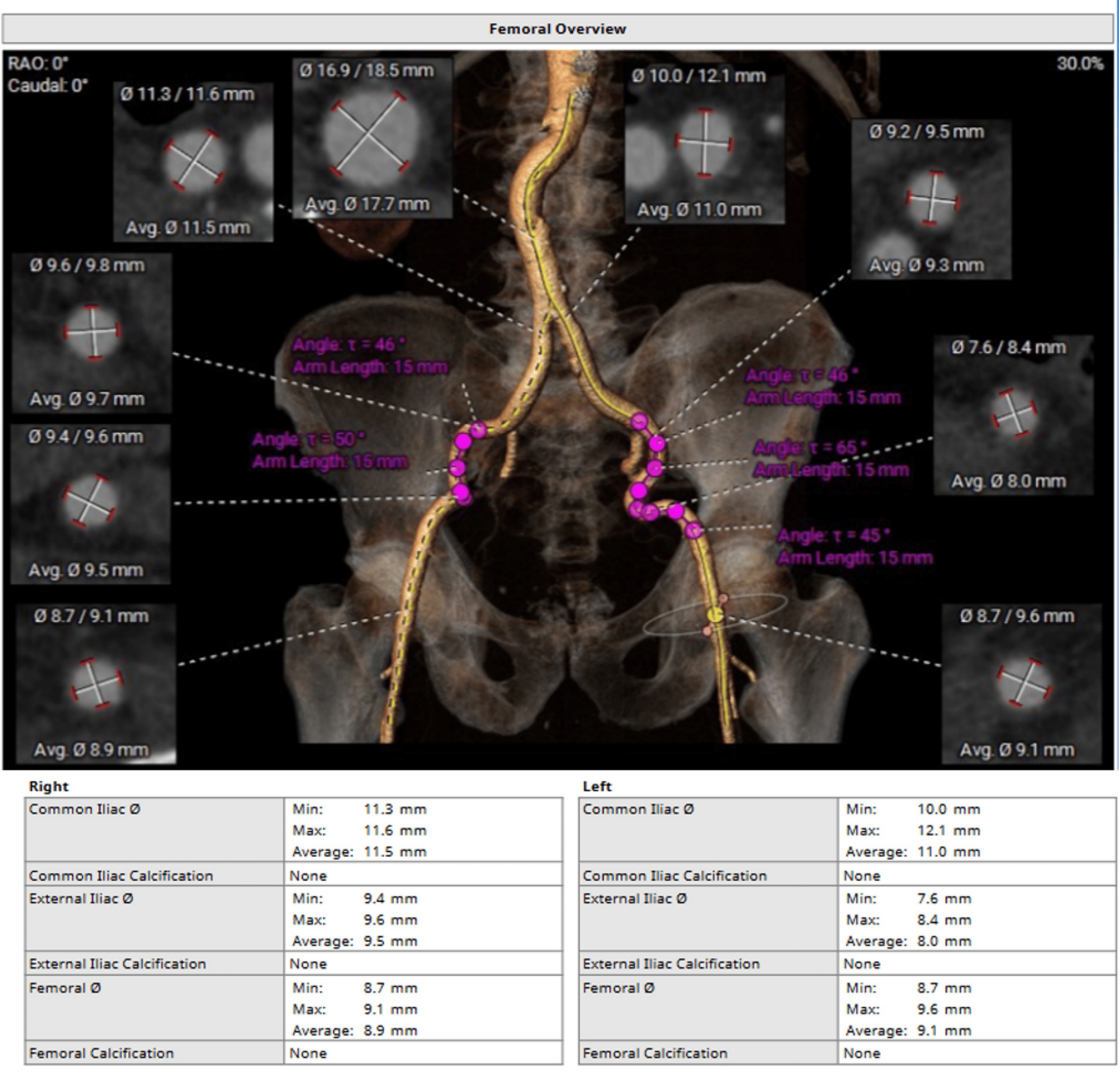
Femoral overview from computed tomography angiography for TAVR The abdominal aorta showed an adequate caliber, with no evidence of aneurysmal dilation or significant atherosclerotic plaques. The iliac and femoral arteries presented sufficient diameter for device insertion, with minimal calcifications and no relevant stenosis.
